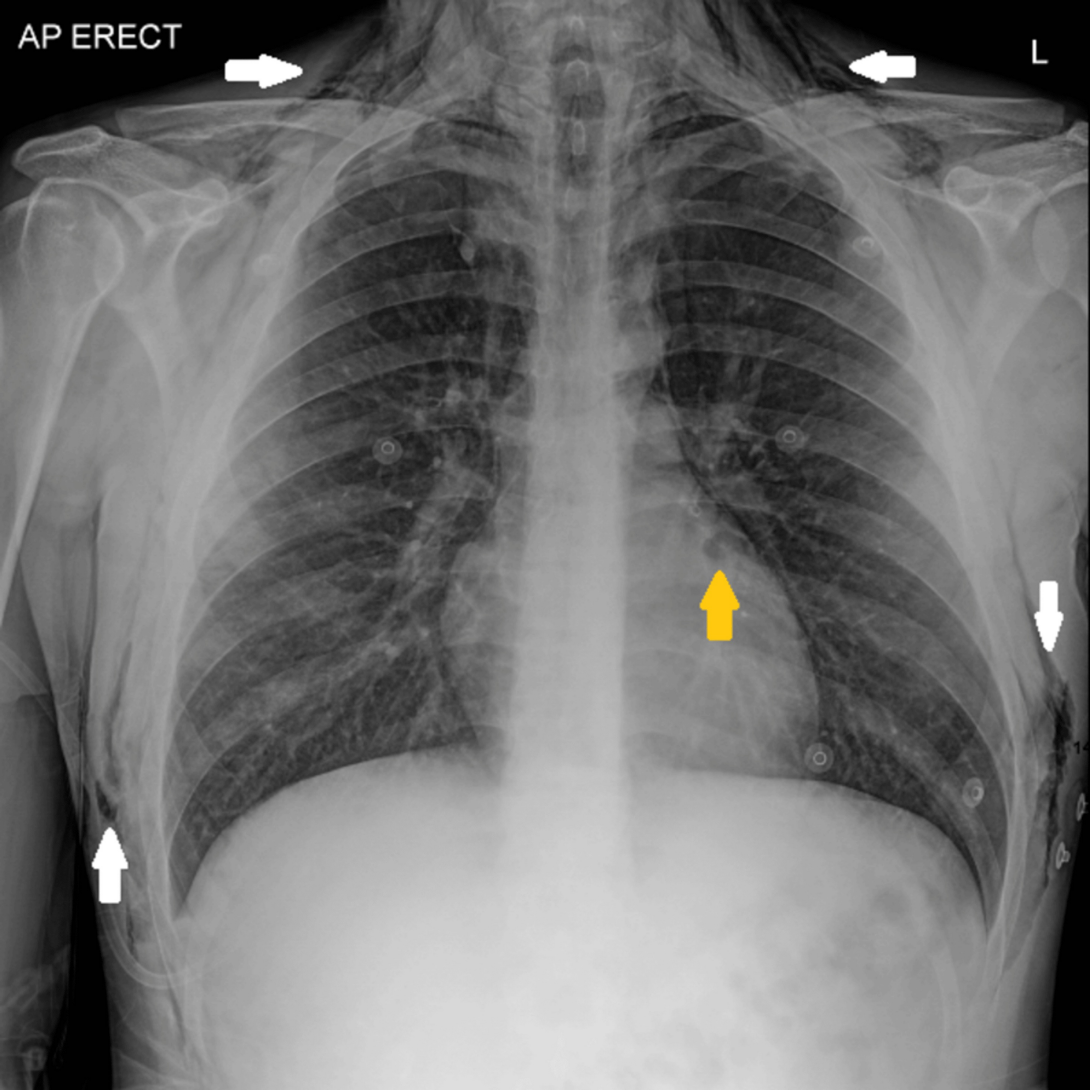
X-ray of the chest demonstrating extensive surgical emphysema (white arrows) in the chest wall and subcutaneous tissues, extending up to the neck on both sides, and associated pneumomediastinum (yellow arrow).
